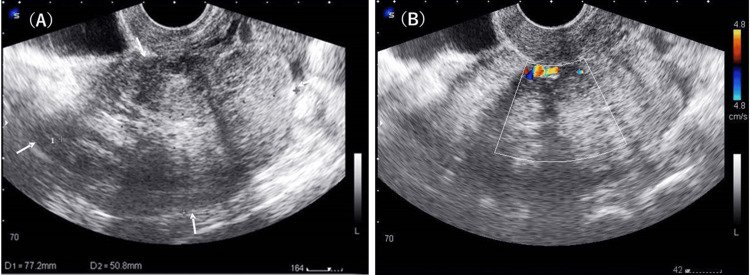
Transvaginal ultrasonography Transvaginal ultrasonography revealed a large, well-circumscribed solid mass (77 × 50 mm) in the left adnexa (A, arrows). This mass showed internal vascularity on colour Doppler imaging (B).
